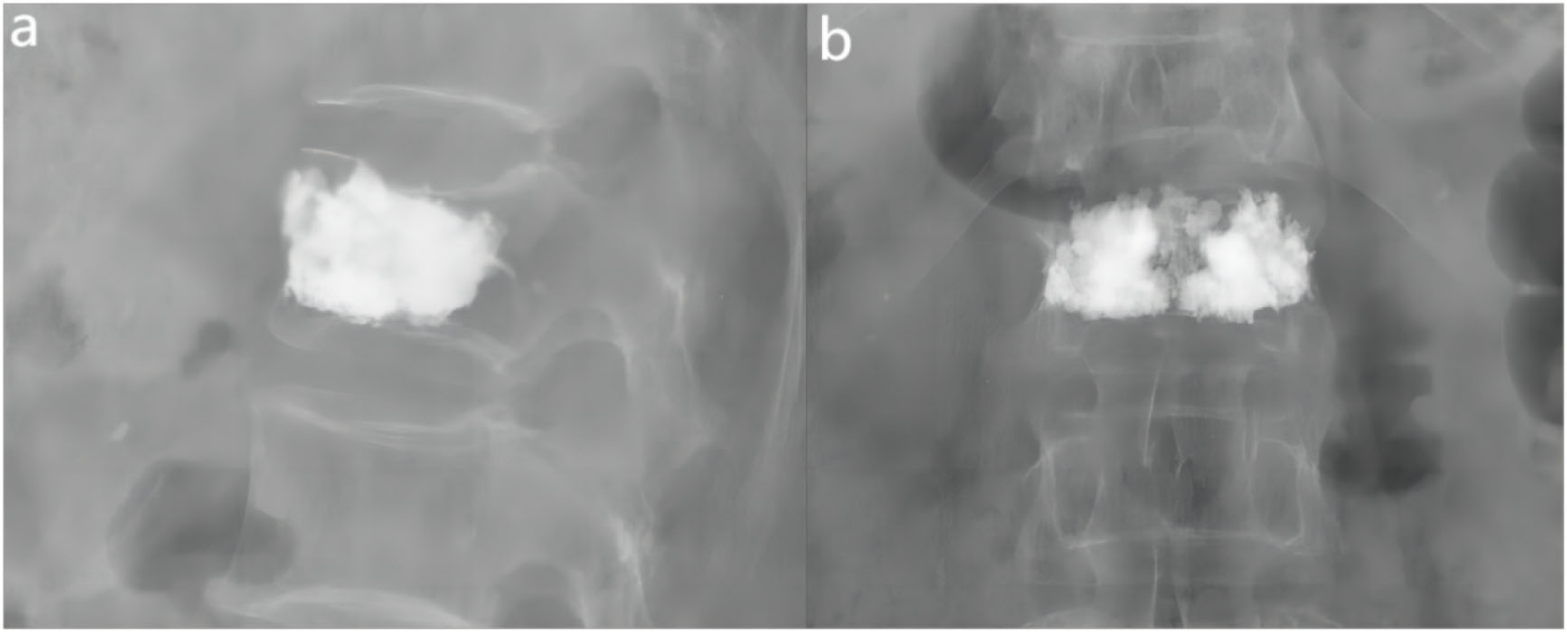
Examples of anterior-lateral radiographs after surgery for fractured vertebrae. (A) The lateral X-ray view showed that the distribution of bone cement reached the anterior and posterior edges of the vertebral body. (B) The ortho-X-ray view showed the distribution of bone cement near the upper and lower endplates.
